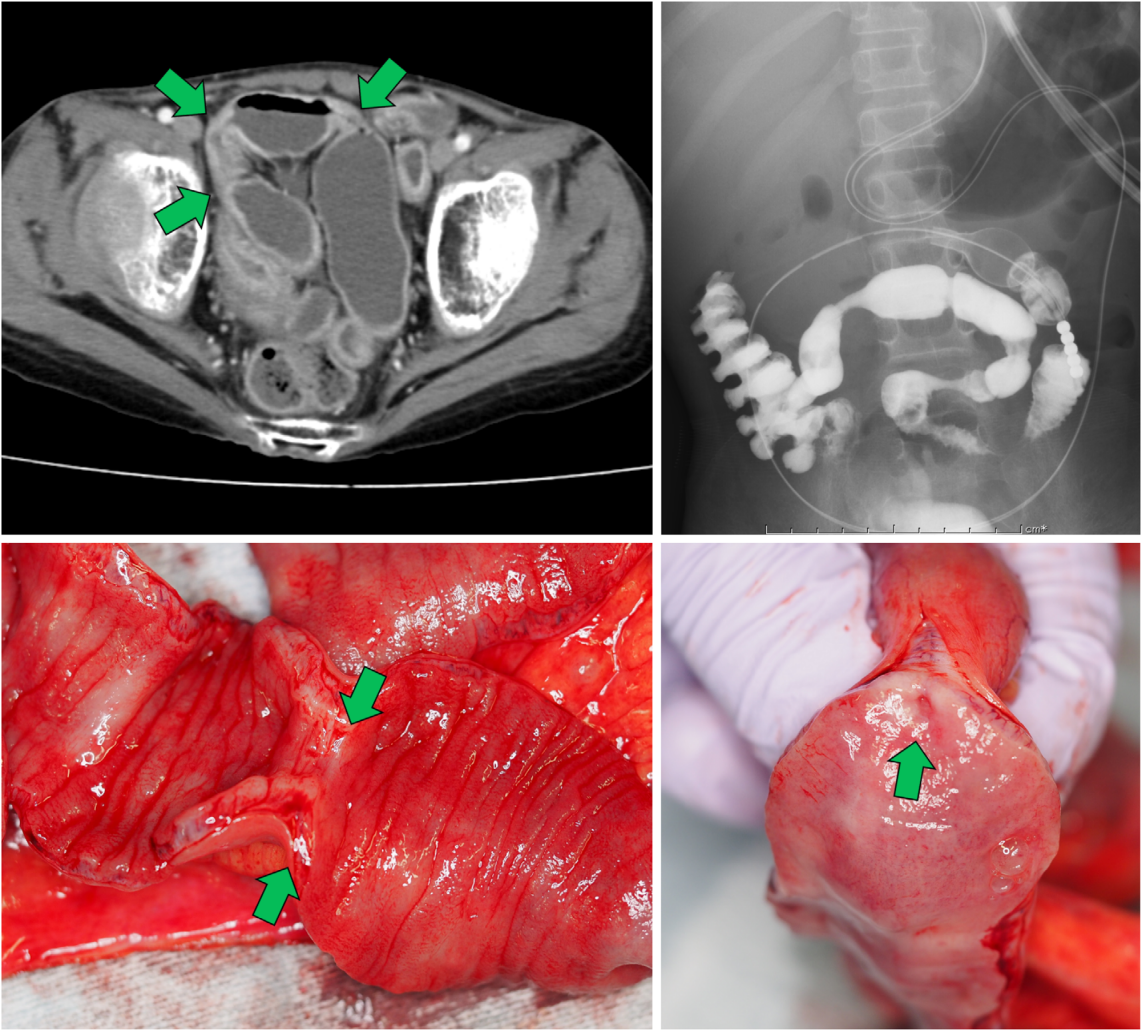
(top) Multiple strictures of the ileum were observed on contrast‐enhanced X‐ray imaging and computed tomography (bottom) Multiple strictures of the ileum were grossly confirmed at operation
